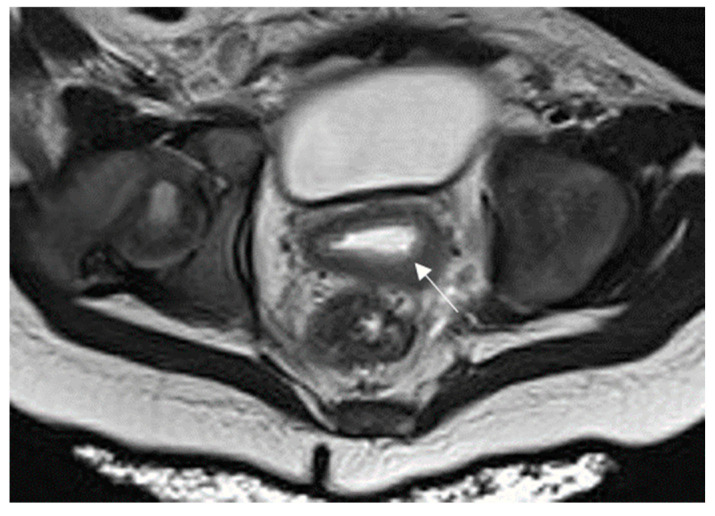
Cervicitis in a 16-year-old female presenting with acute pelvic pain after inconclusive US. Axial T2-weighted image showed cervical enlargement with stromal hyperintensity and wall thickening (arrow). MRI findings, in combination with clinical and laboratory evaluation, supported the diagnosis of cervicitis.
